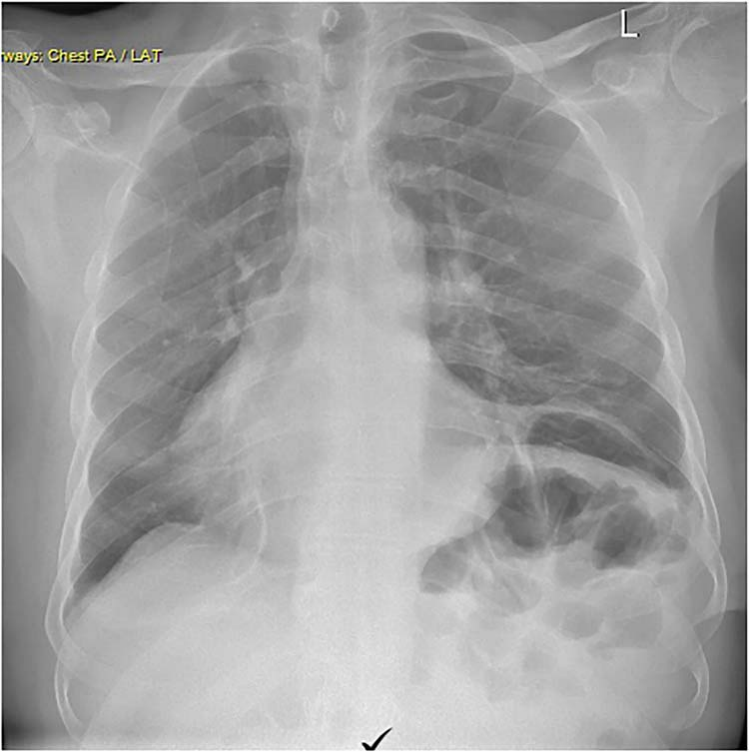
Chest X-ray performed 2 years post-procedure.
